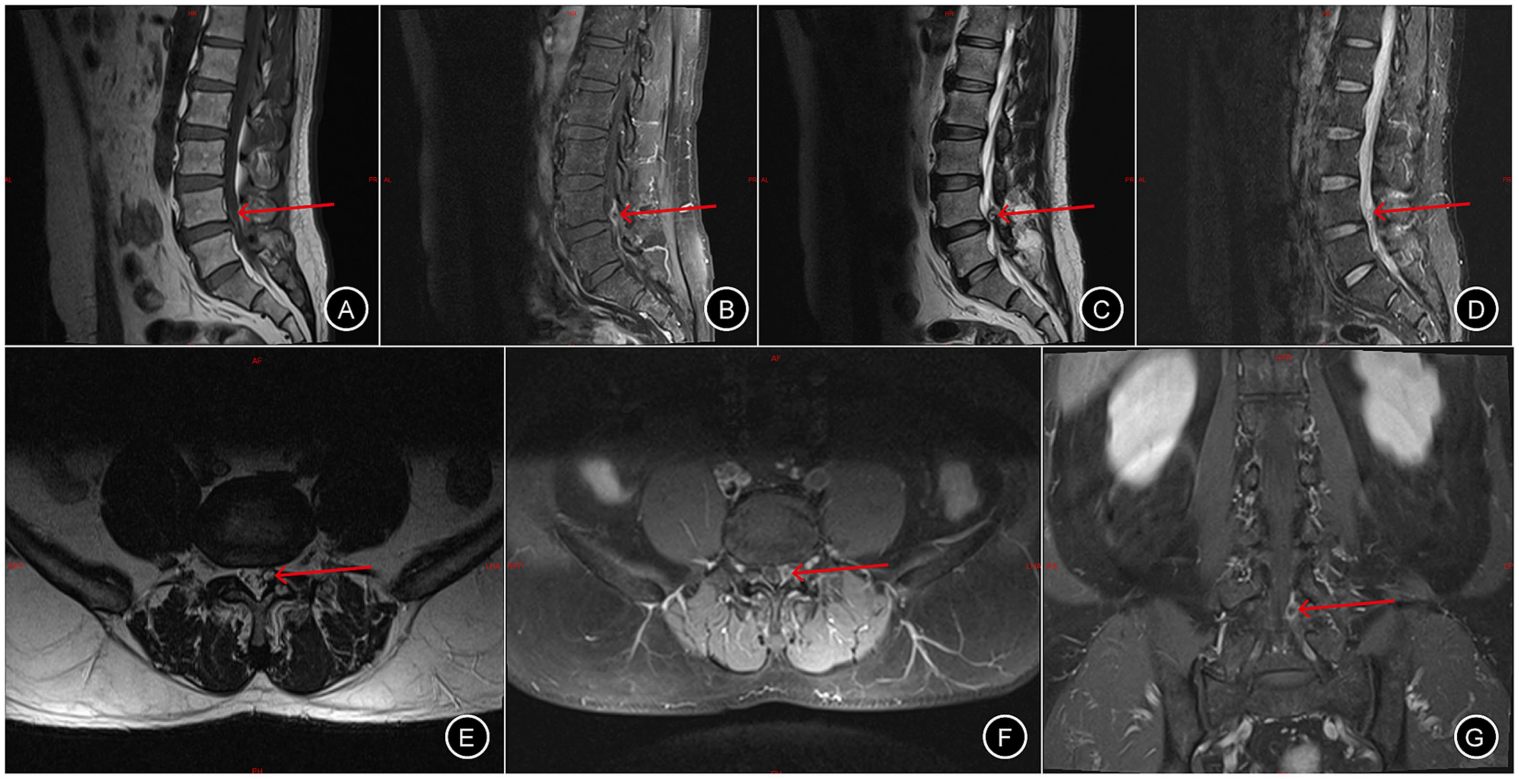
Lumbar intervertebral disk MRI with and without contrast. Panels (A–G) depict the sagittal T1-weighted, sagittal T1-weighted post-contrast, sagittal T2-weighted, sagittal T2-weighted fat-suppressed, axial T1-weighted, axial T1-weighted post-contrast, and coronal T1-weighted post-contrast, respectively. The red arrow indicates the schwannoma.
